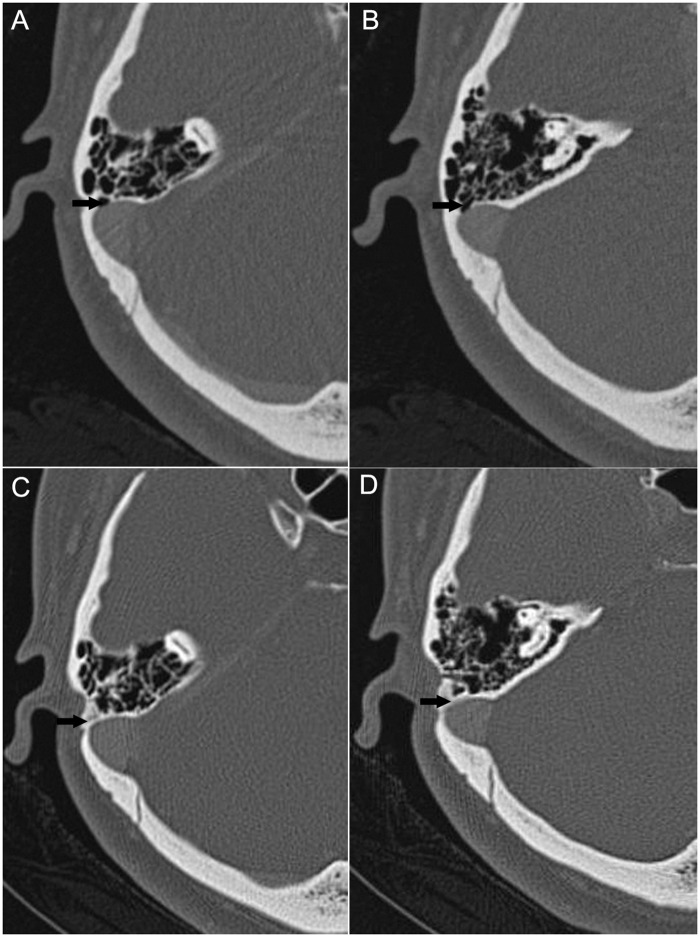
Preoperative (A, B) and postoperative (C, D) CTA findings in a patient (Patient 13) with complete resolution of PT following surgery. The layers in (A) and (B) correspond to those in (C) and (D), respectively. The dehiscences are completely repaired in the two layers postoperatively (arrowheads).
